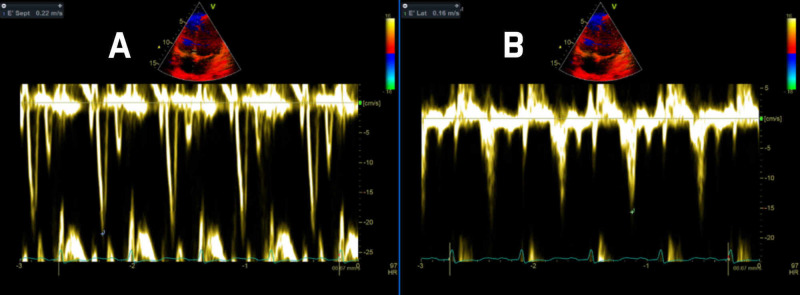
Classic annulus inversus suggestive of the physiology of constrictive pericarditis. (A) Tissue Doppler pulsed wave over septal annulus of the mitral valve. (B) Tissue Doppler pulsed wave over lateral annulus of the mitral valve.
